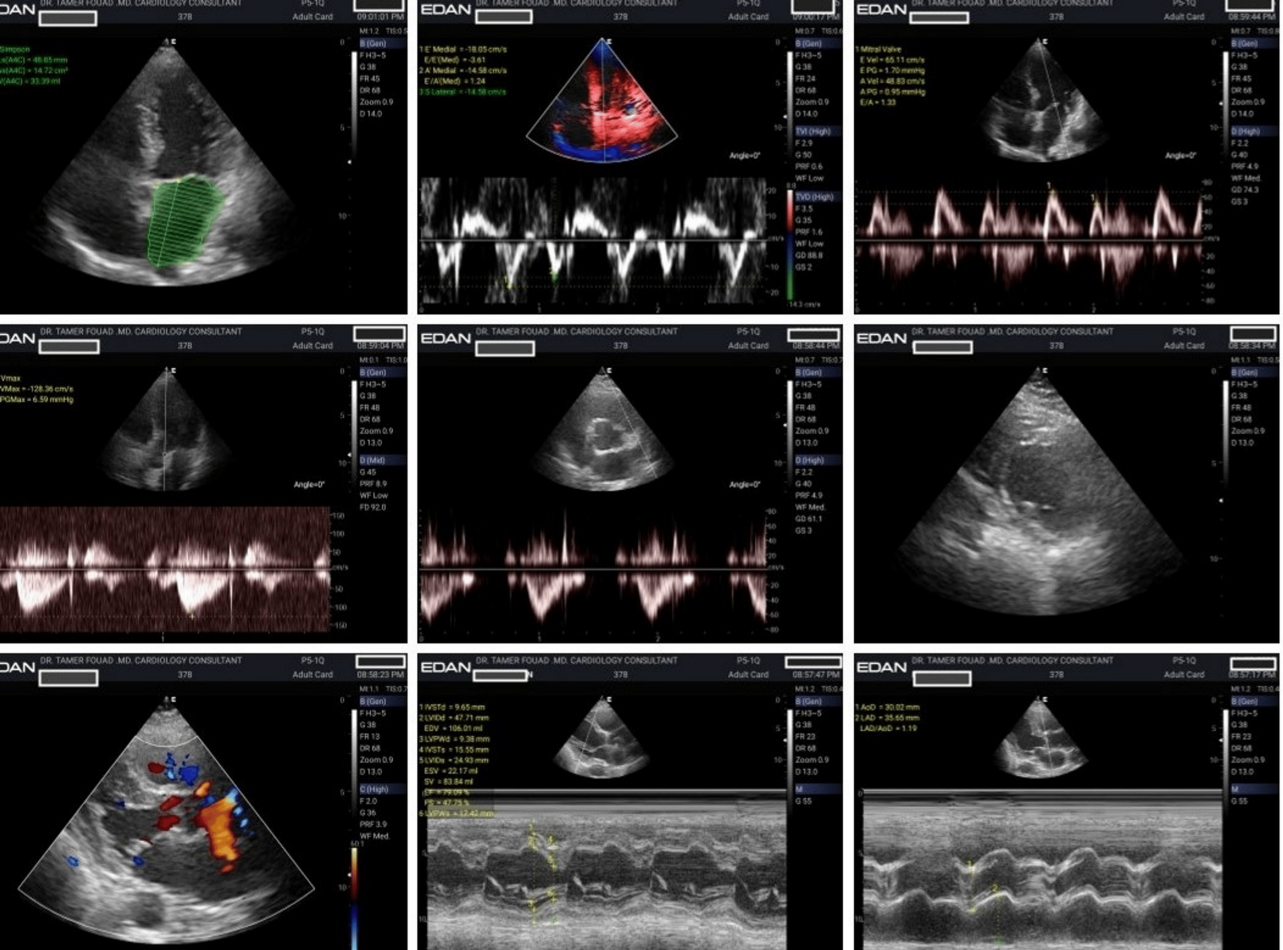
Improvement in echocardiographic parameters after SGLT2 inhibitor treatment SGLT2: sodium-glucose cotransporter 2 This figure shows an improvement in left atrial size and diastolic function after SGLT2 inhibitor therapy. A follow-up study shows a noticeable reduction in left atrial dimension and volume compared to baseline. The mitral inflow pattern and tissue Doppler indices also show a shift from a restrictive physiology towards a normal pattern, consistent with improved left ventricular compliance and lower filling pressures
